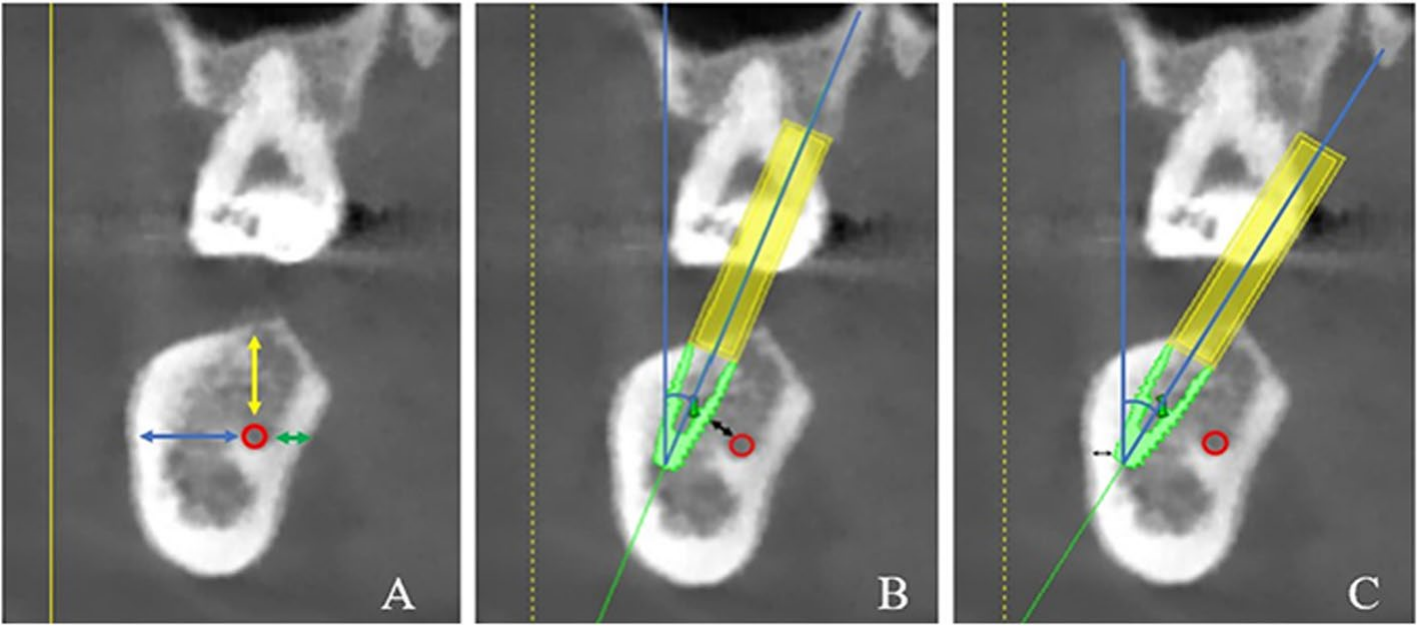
Cone beam computed tomography. A Measurement of the distance from the mandibular nerve canal (red circle) to the buccal cortex (blue arrow), the lingual cortex (green arrow) and the alveolar crest (yellow arrow). B and C Measurement of the minimum (B) and maximum (C) angles of buccal-lingual inclination of the virtual implant
